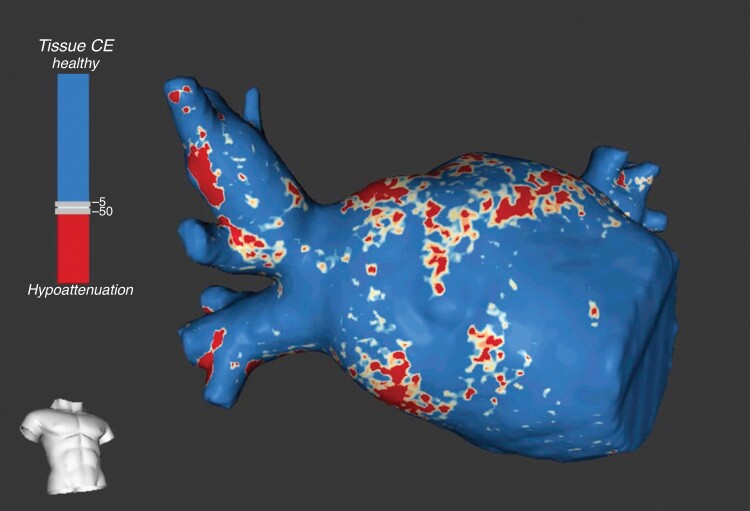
MDCT-derived 3D inFAT map. Septal view of a LA 3D inFAT map. Red areas correspond to dense inFAT (−194 to −50 HU), yellow-orange areas to fat-myocardial admixture (−50 to −5 HU), and blue areas do not harbour inFAT at all (>−5 HU). MDCT, multidetector computed tomography; inFAT, intramyocardial fat; LA, left atrial; HU, Hounsfield unit.
